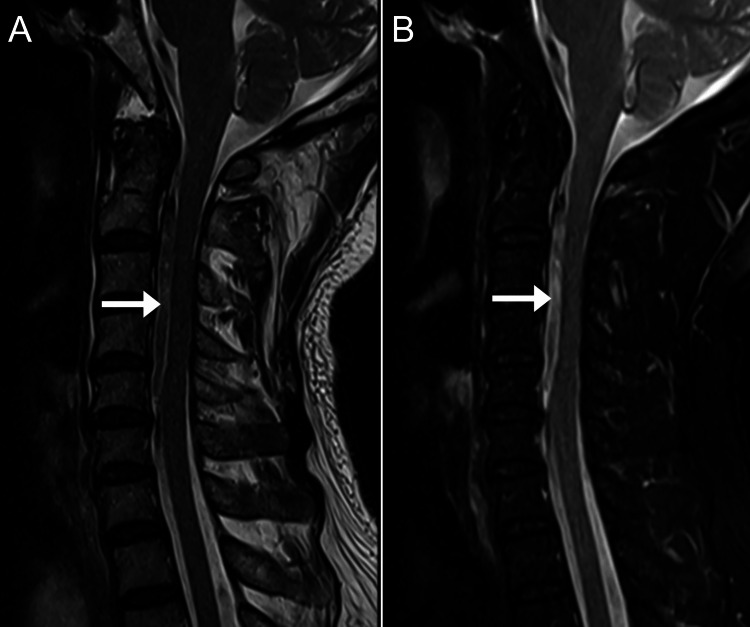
Magnetic resonance imaging of the cervical spine, mid-sagittal T2-weighted images A: A heterogeneously hyperintense and hypointense signal layer in the anterior epidural space (arrow). B: In the T2 fat-suppressed sequence, this layer is mildly hyperintense (arrow).
